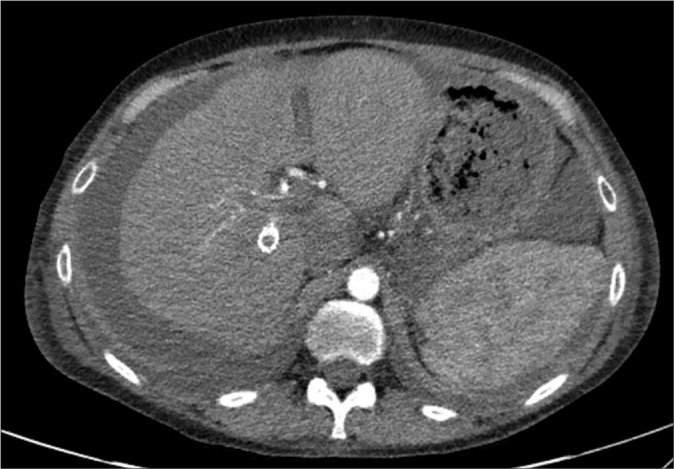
Axial computed tomography angiography performed the day following thrombin injection confirms complete thrombosis of the pseudoaneurysm which is no longer visible.
